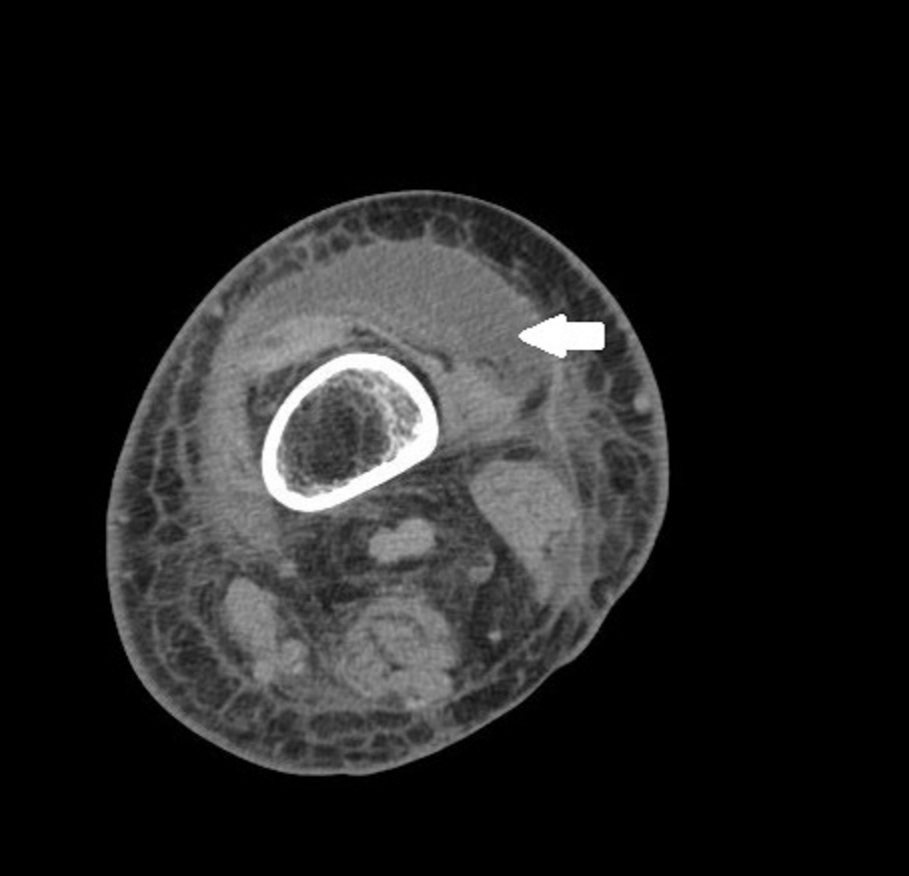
Axial CT Scan of Left Lower Extremity Arrowhead demonstrates discrete intramuscular fluid collection with the largest focus centered in the vastus lateralis.
